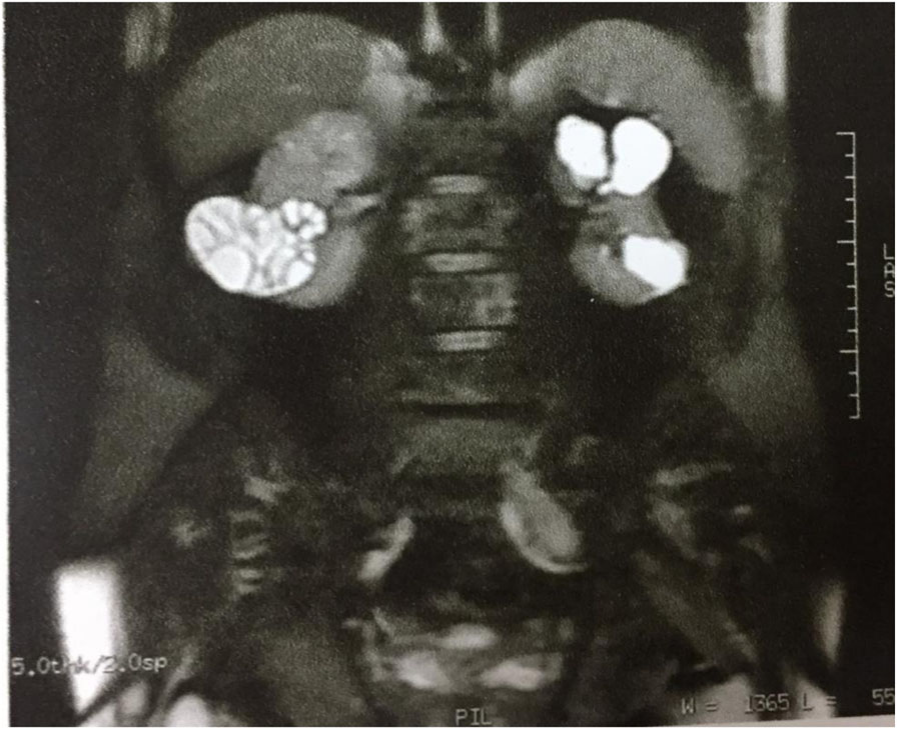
MRI image of the patient on initial admission at our center showing multilocular cyst at right kidney
